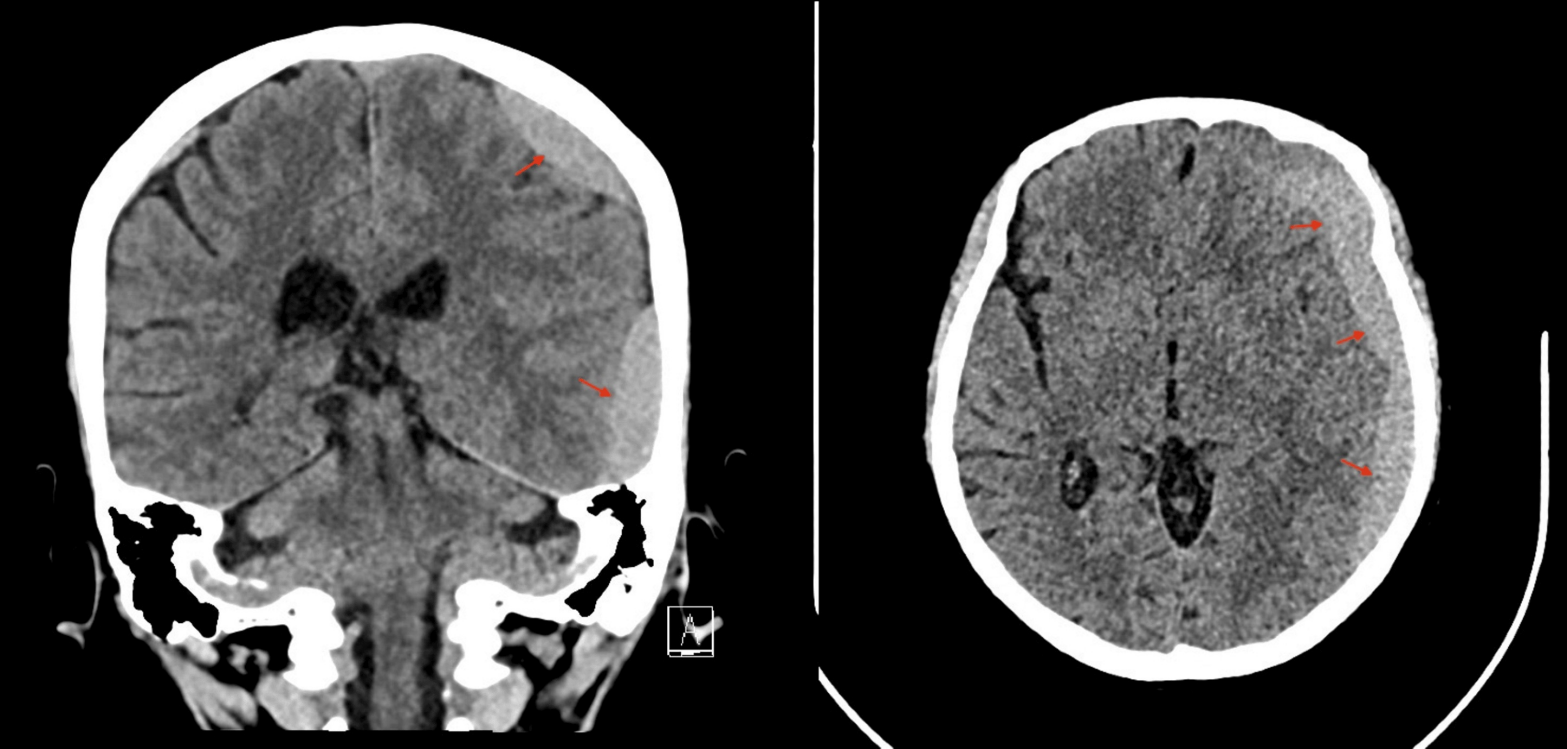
Second admission brain CT pre-MMA embolization. Axial and coronal brain CT scans showing an interval increase in the size of the left cerebral convexity subdural hematoma, with a maximum thickness of 1.7 cm compared to the previous 1 cm. Interval development of a minimal midline shift toward the right side, measuring 0.4 cm. MMA: Middle meningeal artery.
